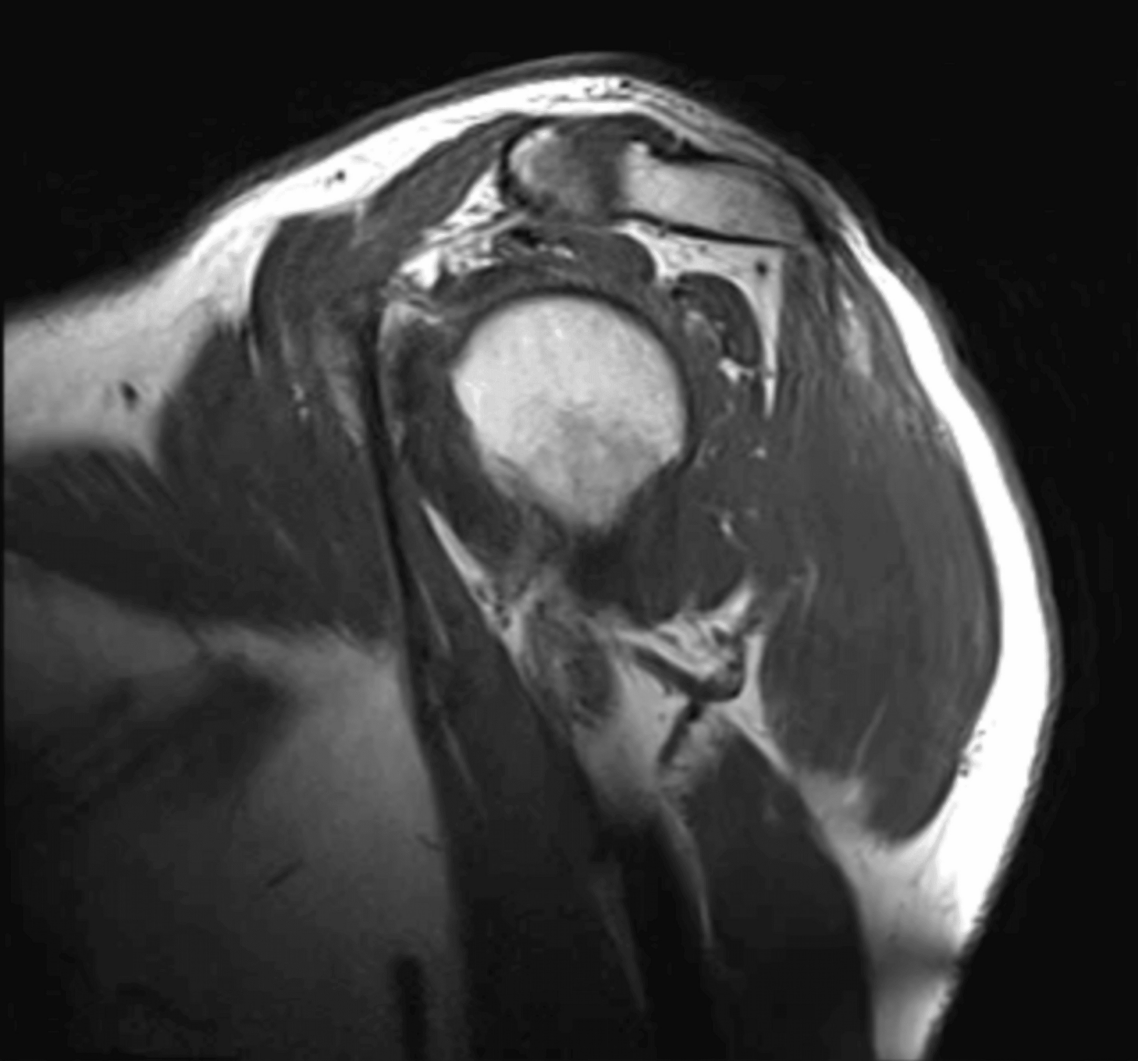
Sagittal T1-weighted magnetic resonance image of the shoulder Sagittal T1-weighted magnetic resonance image of the shoulder girdle demonstrating preserved muscle bulk and homogeneous signal of the peri-scapular and peri-humeral muscles, without edema, fatty replacement, or focal lesions, consistent with absence of active myositis or chronic myopathic changes.
